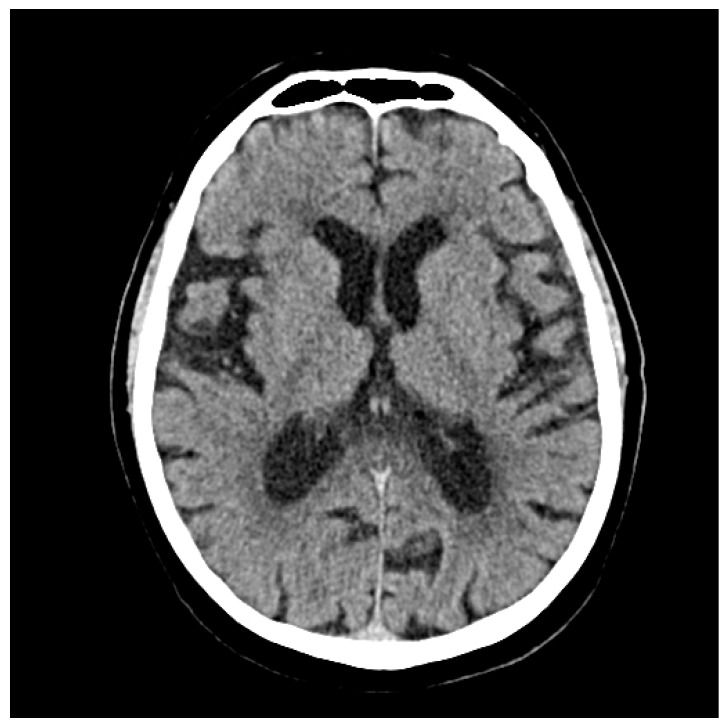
The computed tomography of the brain (September 2023): no acute vascular lesions, no expansive processes visible on the CT scan at the time of the examination.
